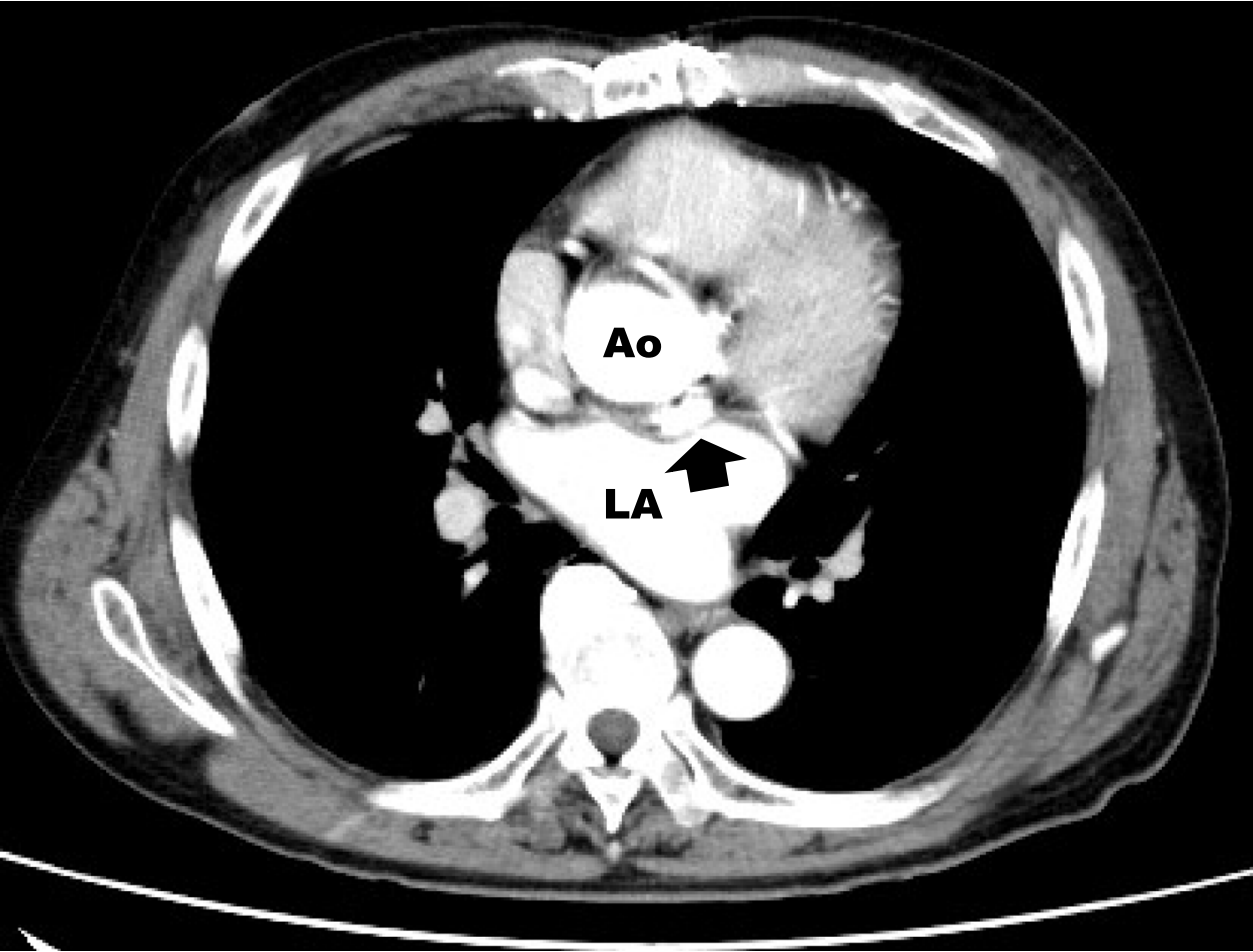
Multidetector computed tomography (MDCT). MDCT showed a pseudoaneurysm (24x10 mm in size, with internal septum) at the dorsal aortic root of the left coronary cusp and noncoronary cusp valve ring (arrow)
